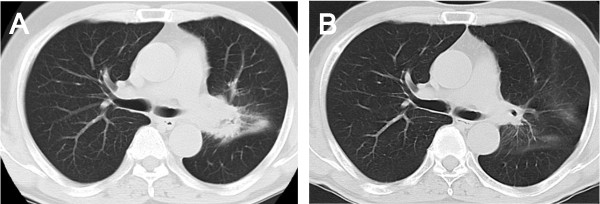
Chest computed tomography images.A: Showing a mass shadow on the left side of the hilum before gefitinib therapy. B: Revealing a good response of the primary tumor after three months of gefitinib therapy
